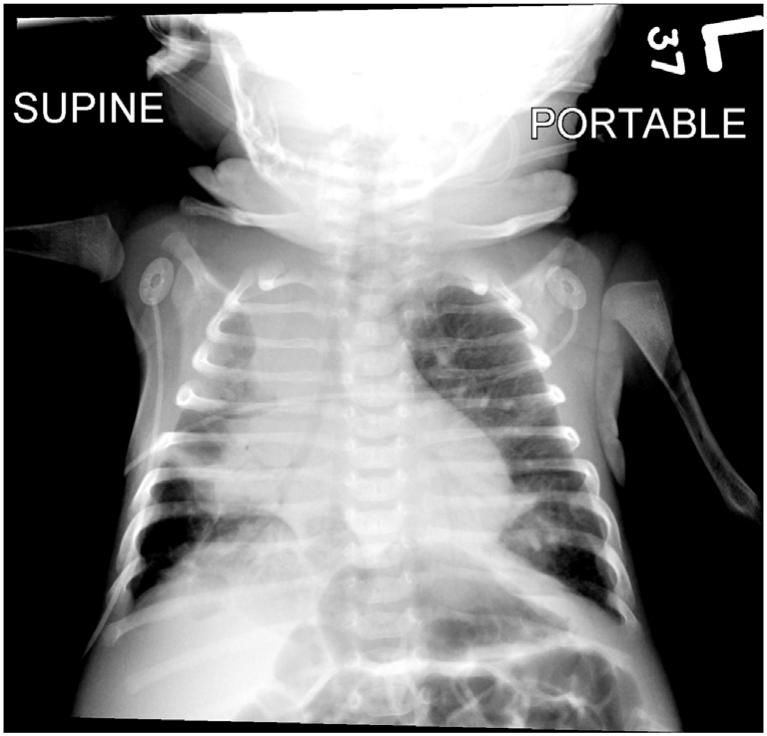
A portable chest radiograph obtained earlier in the day demonstrating bilateral parenchymal opacities.
